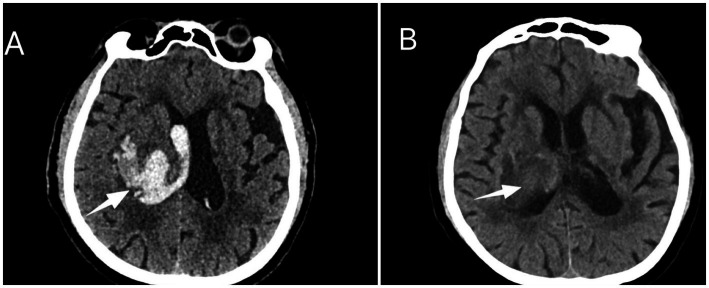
(A) Cranial computed tomography demonstrates a patchy hyperdense shadow in the right thalamus, basal ganglia, and paraventricular region. The lesion measures approximately 3.7 × 3.5 × 3.1 cm at its largest cross-section, with a mean density of approximately 67 Hounsfield Units. It is surrounded by a hypodense rim and exerts mass effect on the adjacent lateral ventricle. Hyperdense material is also observed filling the ventricular system. (B) Cranial computed tomography reveals a patchy mixed-density shadow, containing both hyperdense and hypodense components, located in the right thalamus, right basal ganglia, and paraventricular area adjacent to the right lateral ventricular body. Mild compression of the right lateral ventricle is noted.
